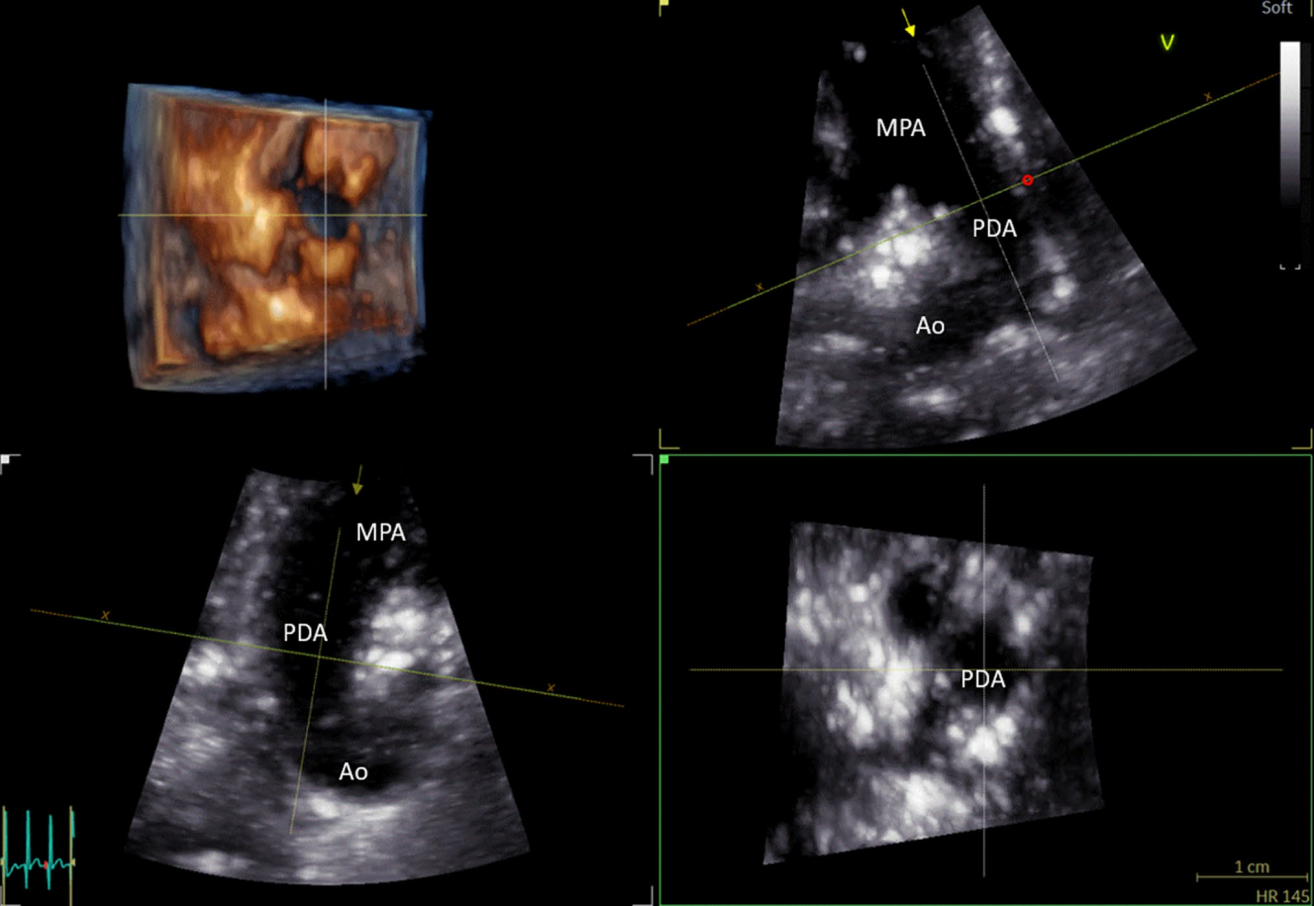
3D image of a large PDA with multiplanar and 3D representation. The 3D rendering of the PDA is seen in the top left corner, demonstrating a “down the tunnel” view of the PDA from the main pulmonary artery to the aorta. The top-right image represents the reference plane of the PDA from a parasternal short axis view. Individual axes have been adjusted to represent the full diameter of the PDA in each view
